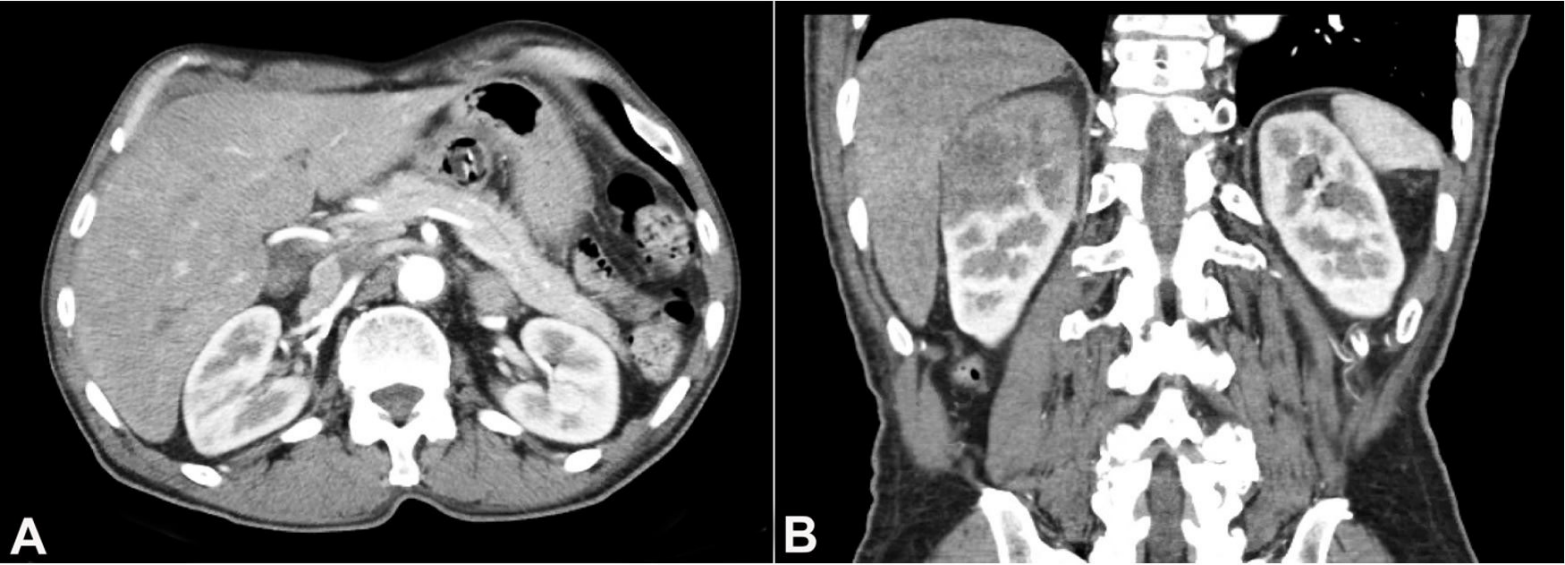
CT scan demonstrating ill-defined low-attenuation masses within the superior pole and mid-region of the right kidney.
